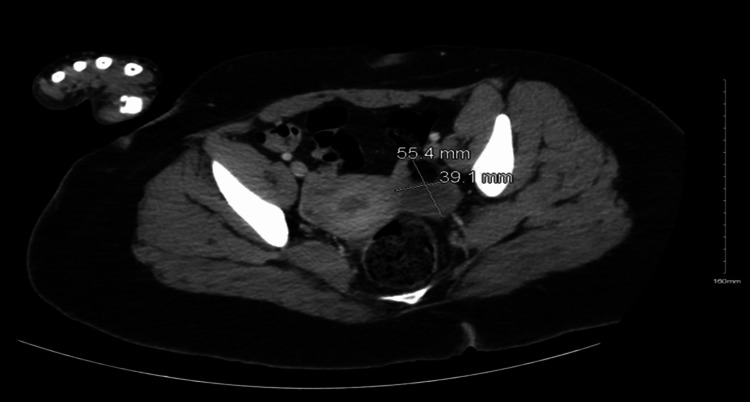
CT scan of the pelvis demonstrating a 55.4 mm by 39.1 mm left ovarian teratoma.
